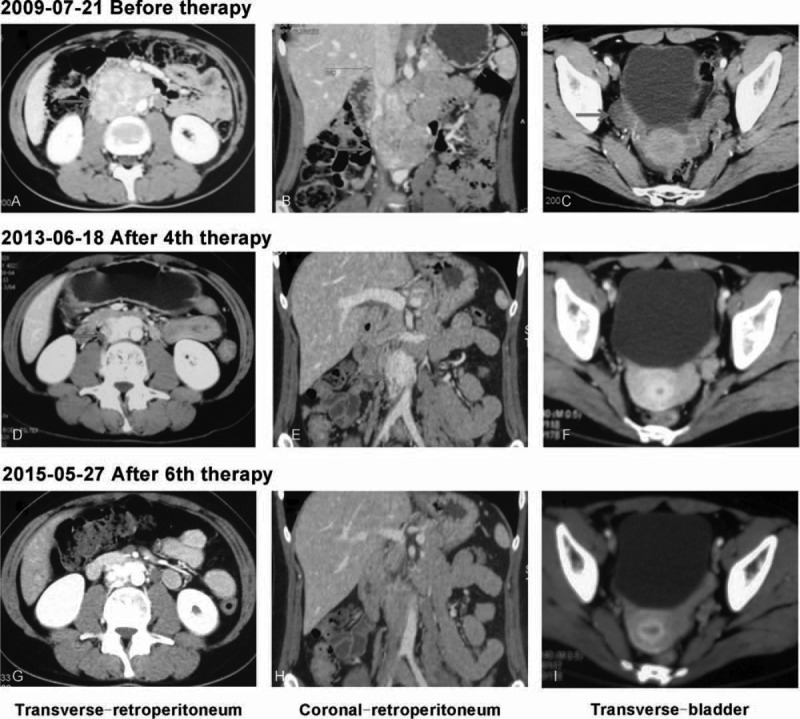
Abdominal computed tomography showed lesion of the retroperitoneum and urinary bladder before and after ^131^I-metaiodobenzylguanidine (^131^I-MIBG) therapy. (A–C) Before ^131^I-MIBG therapy; (D–F) after 4 course ^131^I-MIBG therapy; and (G–I) after 6 course ^131^I-MIBG therapy.
